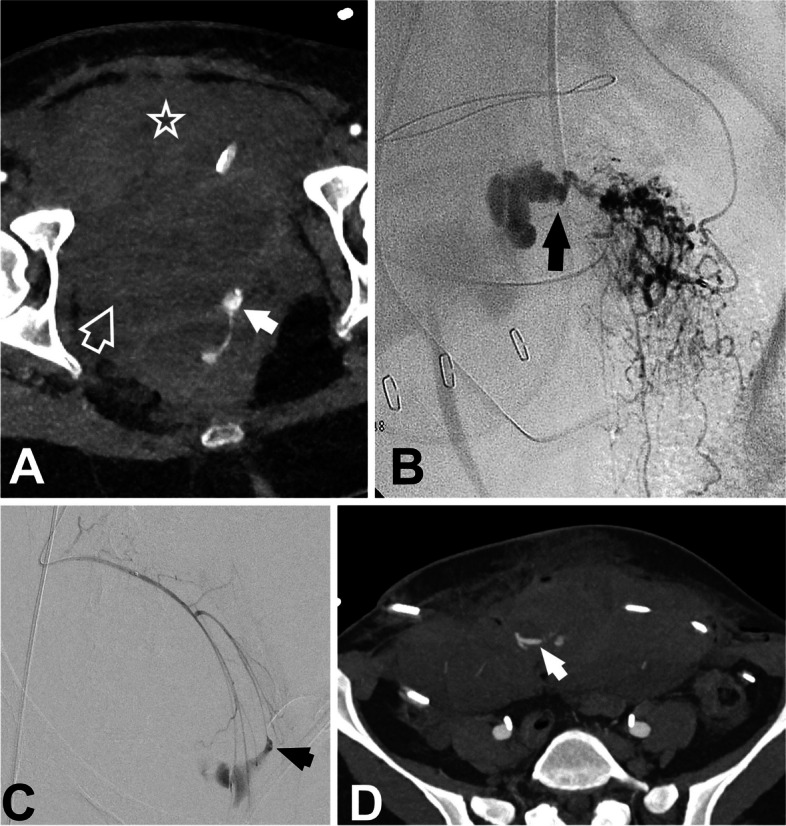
A 36-year-old postpartum patient with hemodynamic instability and a sudden drop in hematocrit in the immediate postpartum period following an instrumental delivery with spatulas, despite the absence of significant vaginal bleeding. Angio-CT with arterial and delayed contrast phase was performed, revealing active extrauterine bleeding at the cervicovaginal level (arrow) with the formation of hematomas in the perivesical (open arrow) and prevesical (hollow star) spaces. B Selective catheterization and angiography in uterine pseudoaneurism rupture after cesarean delivery with active bleeding (arrow). C Selective catheterization and angiography in cervical pseudoaneurism rupture after cesarean delivery with active bleeding (arrow) after forceps-assisted instrumental delivery. D 32-year-old patient in immediate postpartum period after cesarean delivery. A significant increase in abdominal circumference is observed, along with a decrease in hematocrit and signs of hemodynamic instability. Hematoma of the rectus sheath with signs of active bleeding (arrow) was observed in angioCT
